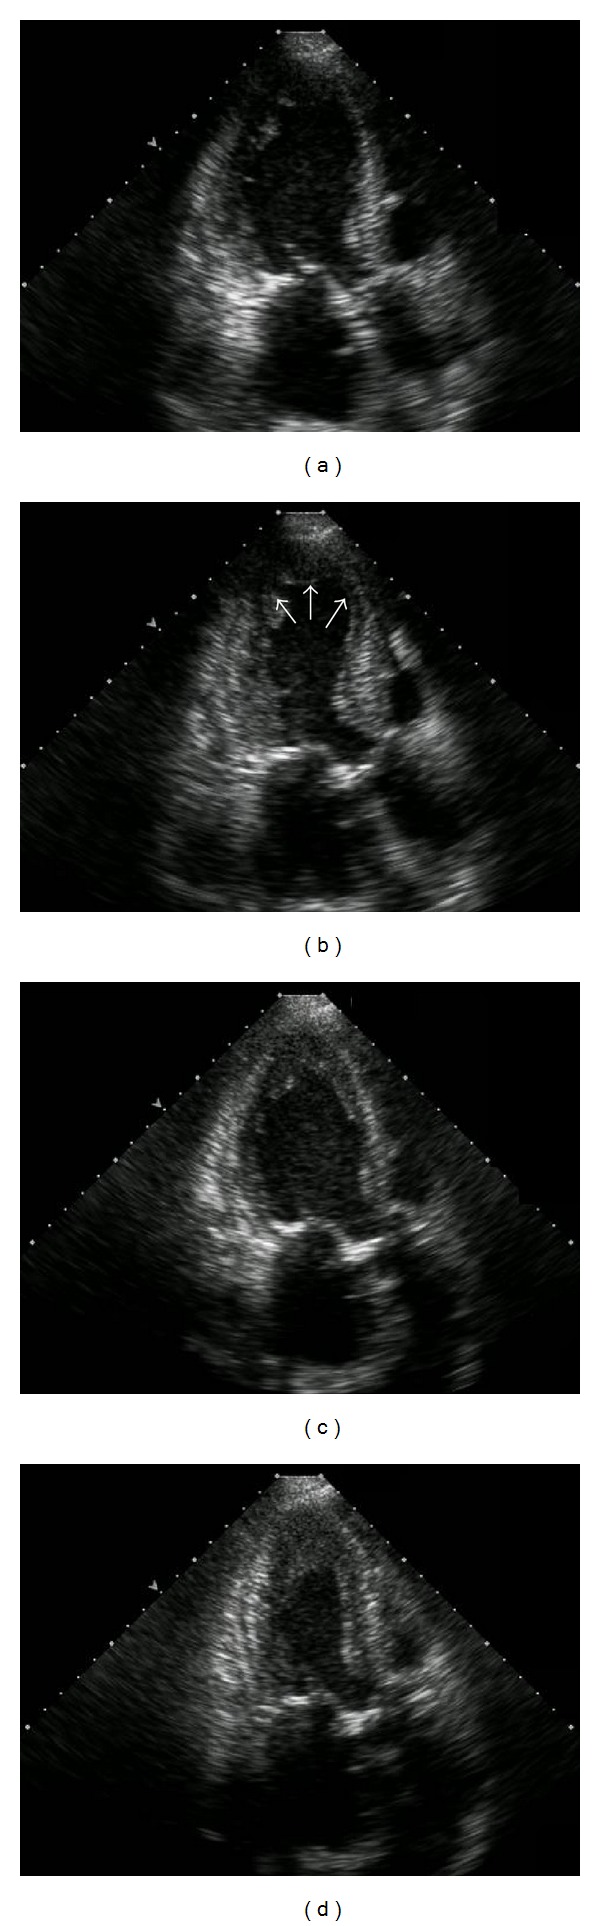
Case 2. ((a), (b)) Echocardiogram on admission demonstrated apical akinesis and ballooning of the left ventricle apex (arrows). (a) End-diastolic phase; (b) end-systolic phase. ((c), (d)) Echocardiogram 3 weeks later showed recovered left ventricular apical wall motion (c) End-diastolic phase; (d) end-systolic phase.
